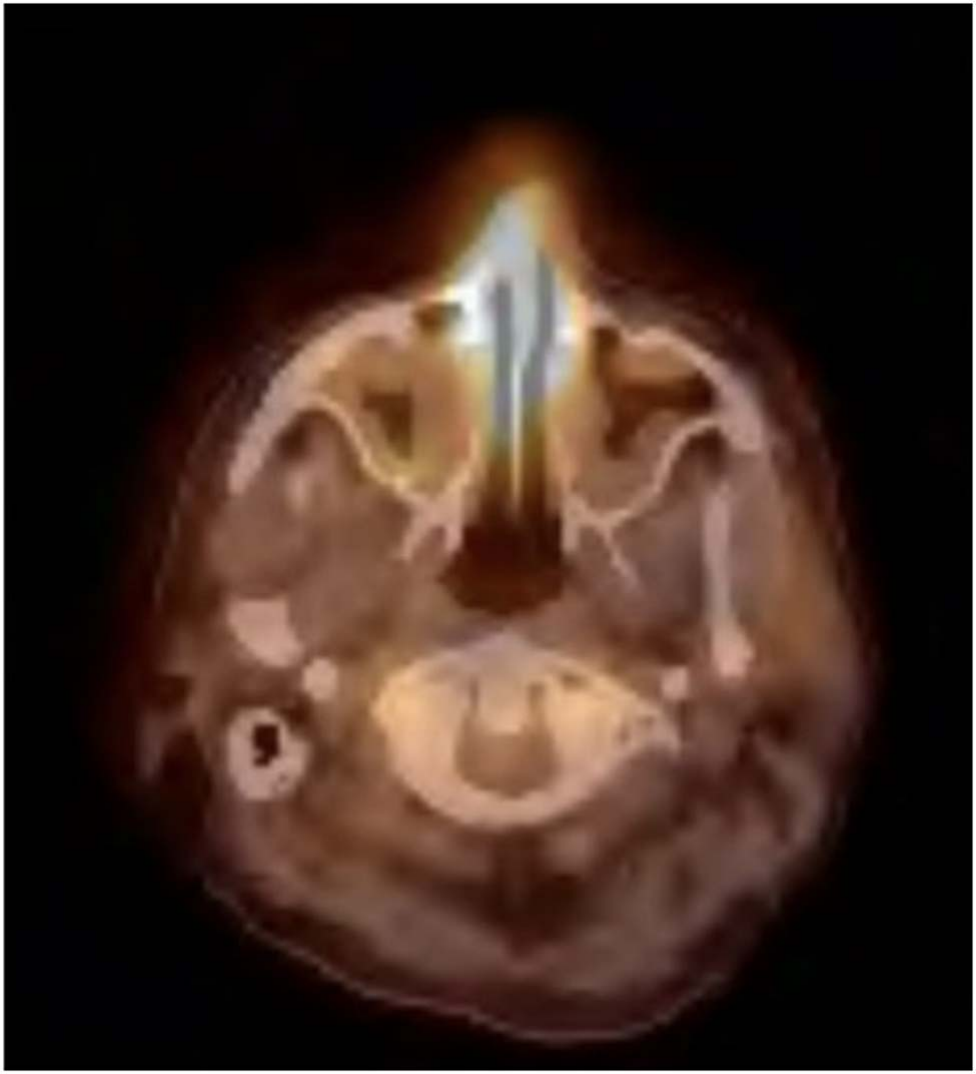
PET-CT of the Skull base: nonspecific moderate increased activity within the nasal cavity in the vicinity of the septum possibly due to inflammation.
